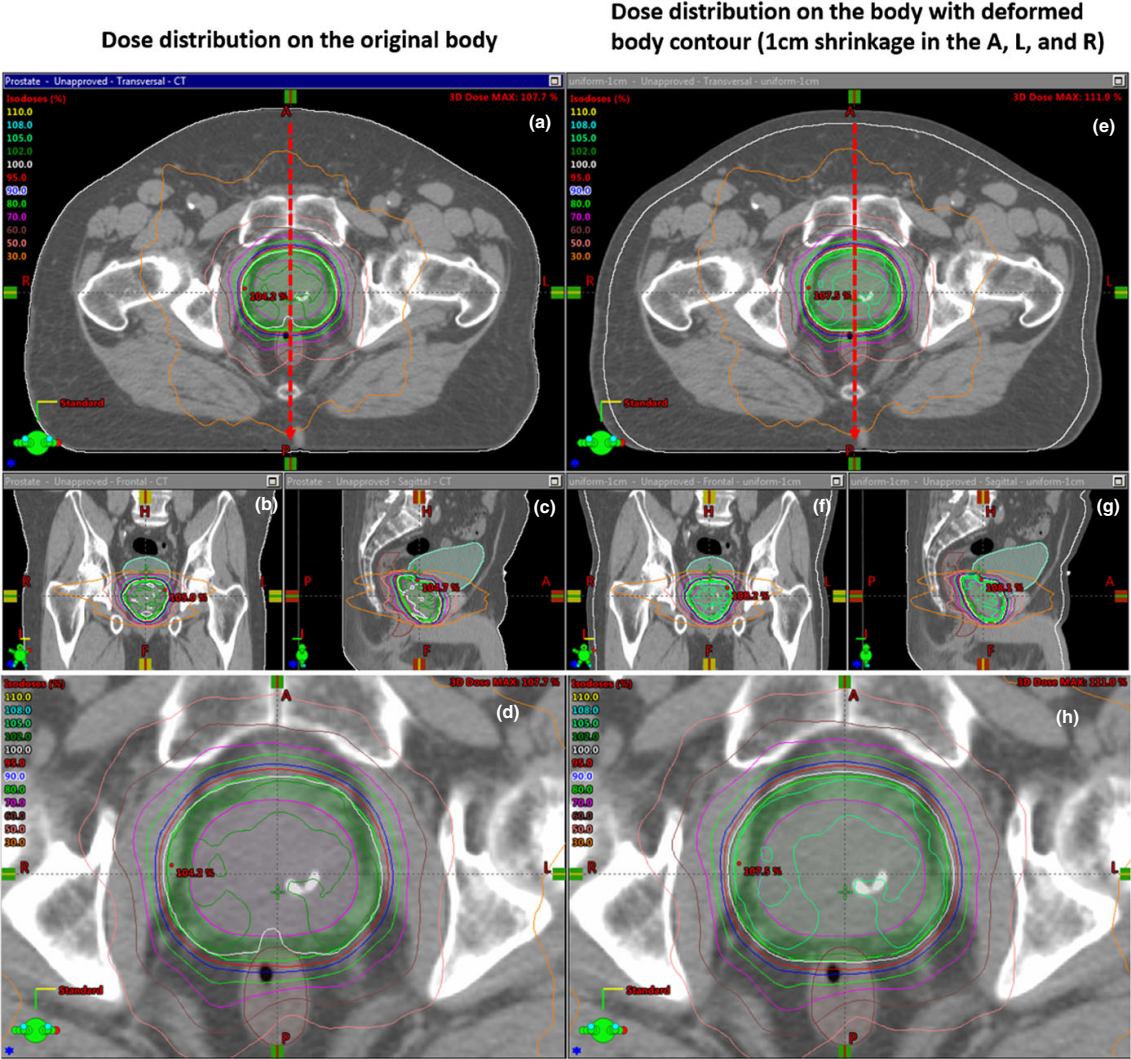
Example dose distributions shown as isodose lines before (a)–(d) and after (e)–(h) external body contour change. The red dashed lines in (a) and (e) represent the direction of the one‐dimensional dose profile chosen. Structures including CTV (magenta), PTV (green), bladder (cyan), and rectum (brown) are contoured in the computed tomography images. (d) and (h) are a magnified view of (a) and (e), respectively.
